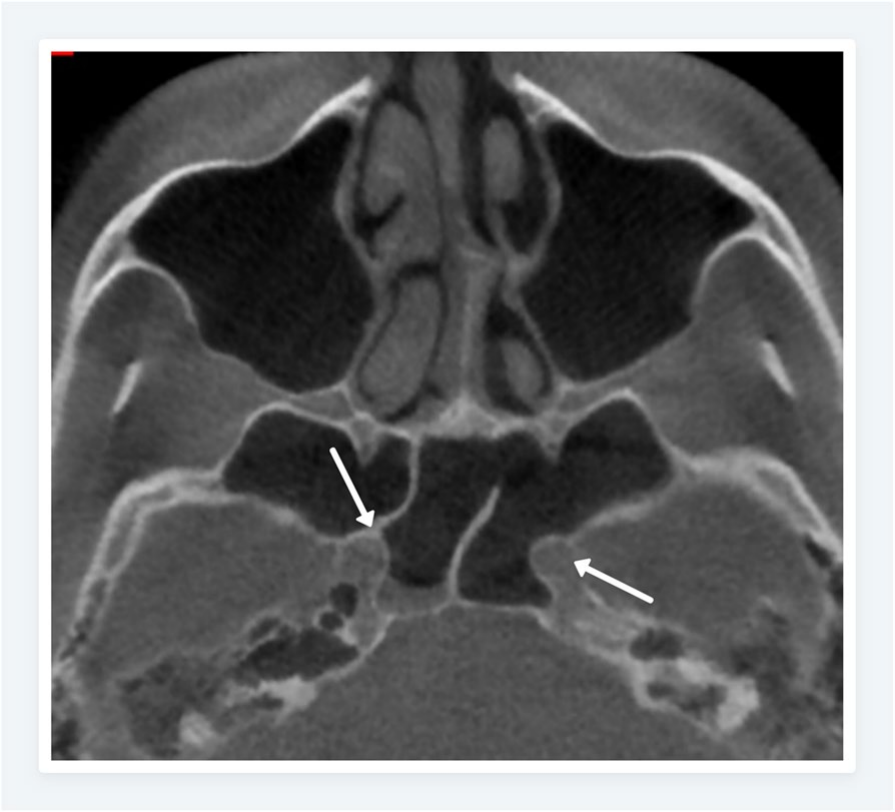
Axial CBCT scan showing bilateral internal carotid artery protrusion (arrows) into the sphenoid sinus with complete accessory septation (on the right side) inserting onto the internal carotid artery bony wall
